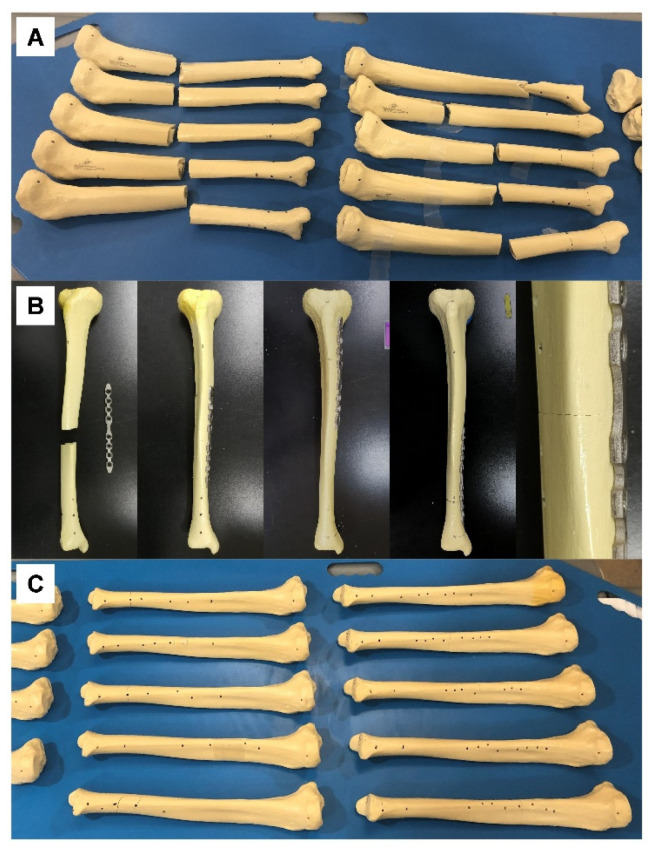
(A) Tibial sawbones fractured at various longitudinal locations of the shaft, prior to CT scan. (B) Sawbones and customized plates prior to fixation (left image in row), fixed at various locations (middle three images in row), and enlarged at the fracture site (right image in row). (C) Tibial sawbones actually fixed with a customized plate based on virtual reduction, prior to CT scan.
